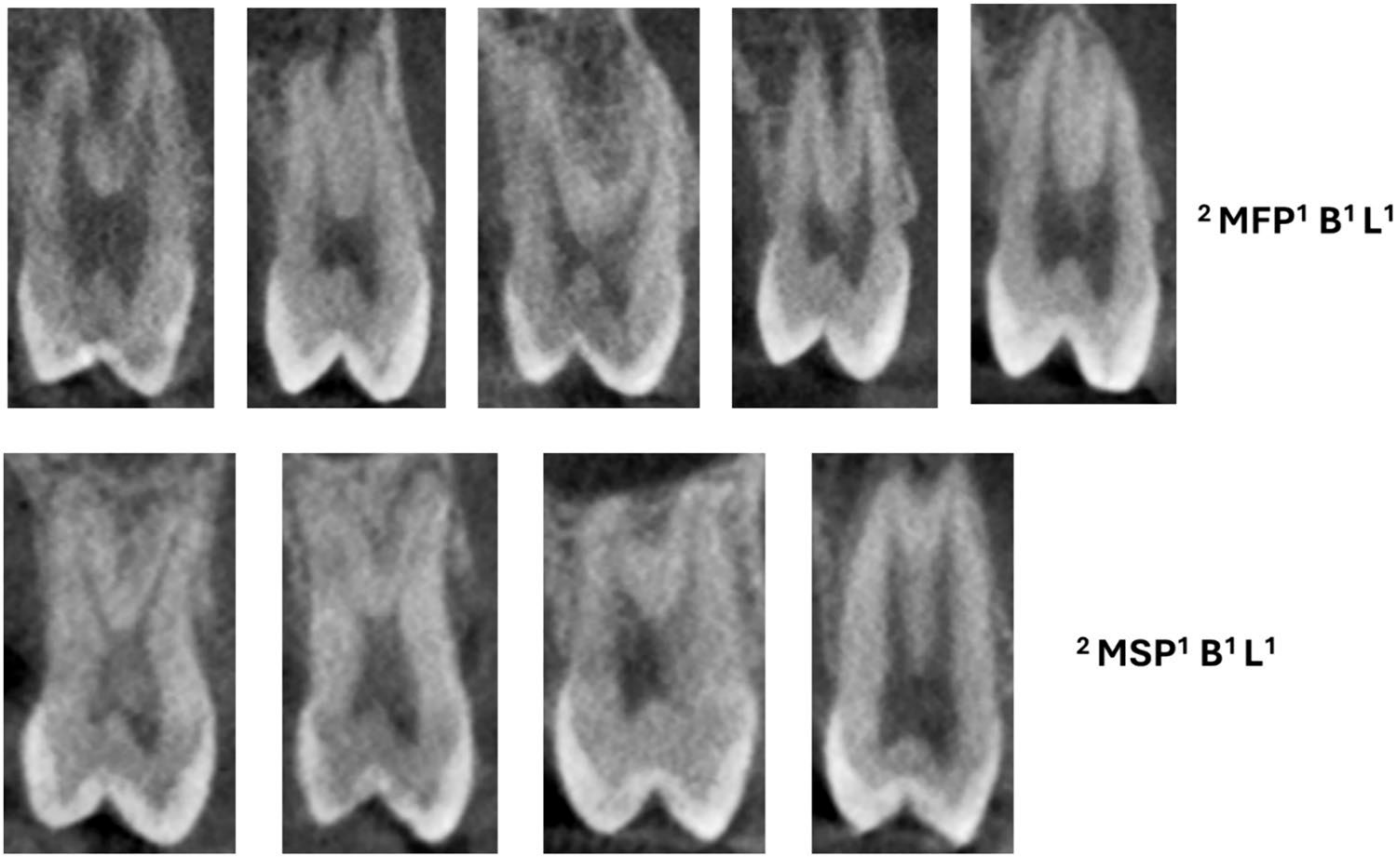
CBCT images (sagittal views) of maxillary premolar (MFP—first premolars, MSP—second premolars) showing root canal morphology with two roots
